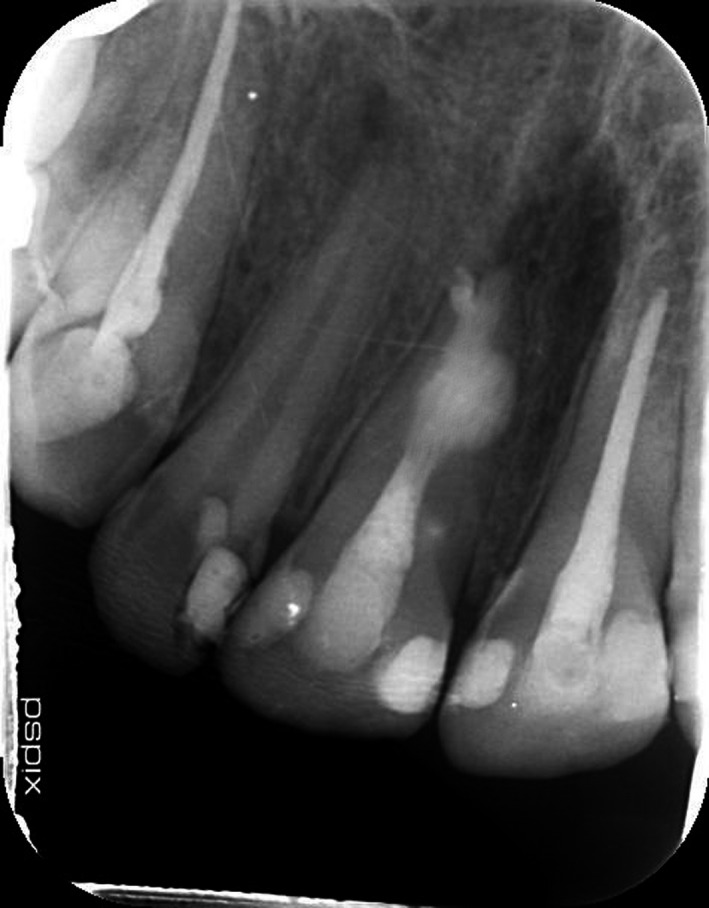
Follow‐up radiograph after 6 mo
